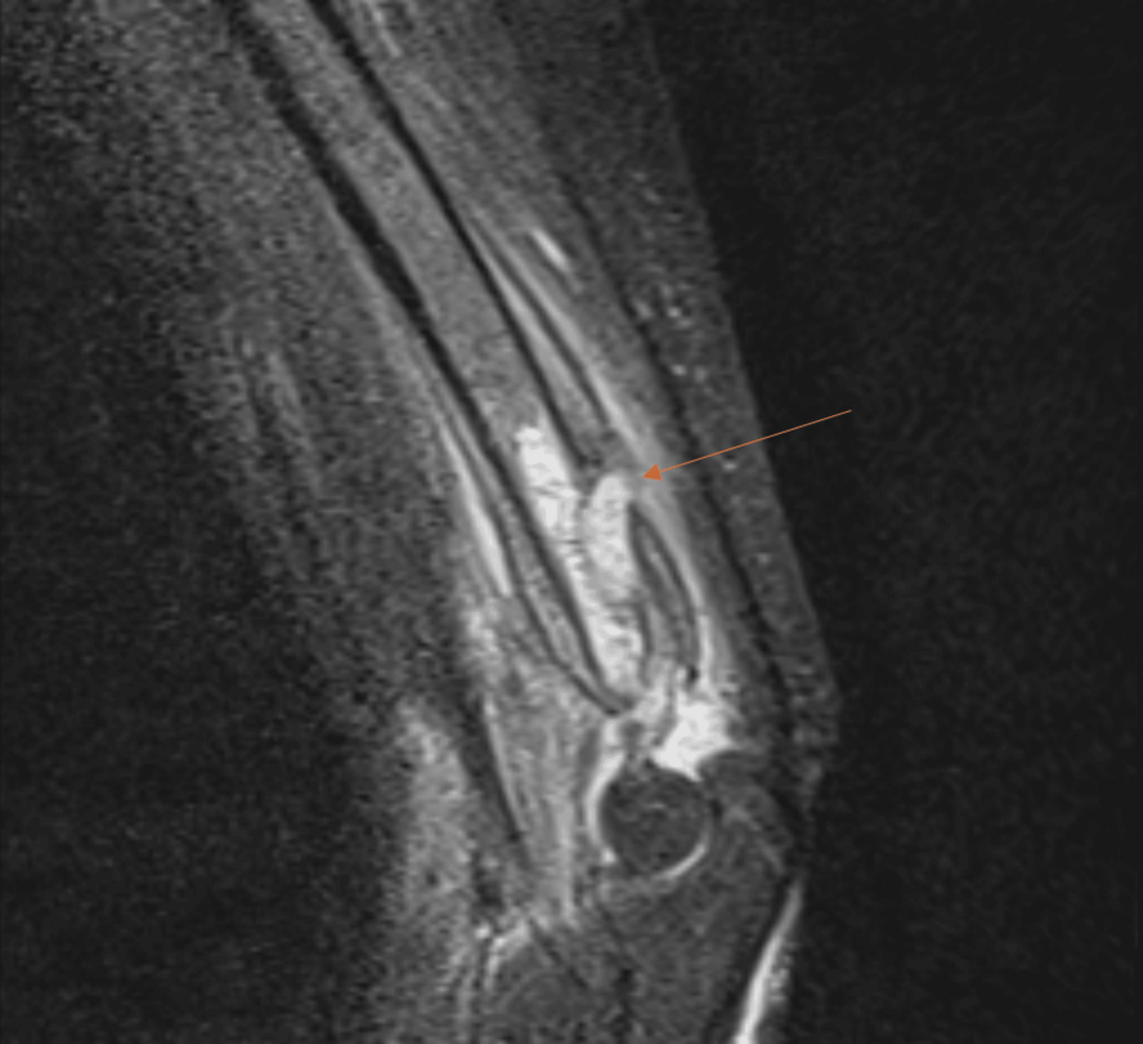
MRI shows a deep bone abscess and posterior sinus Deep bone abscess is shown with the arrow.
